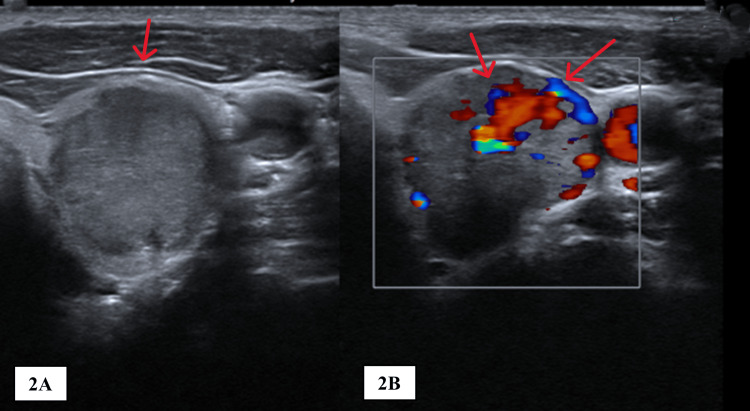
Ultrasound scan of the thyroid showing a nodule (A, arrow), a nodule with increased vascularity (B, arrows).
